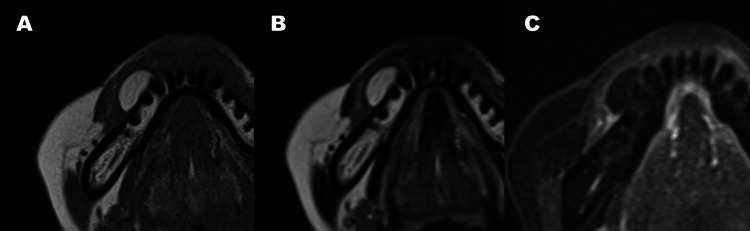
MRI findings T1-weighted image(A) and T2-weighted image(B) showing a homogeneous high-intensity signal corresponding to the mass. STIR image (C) showing a low signal intensity area consistent with fatty tissue characteristics.
